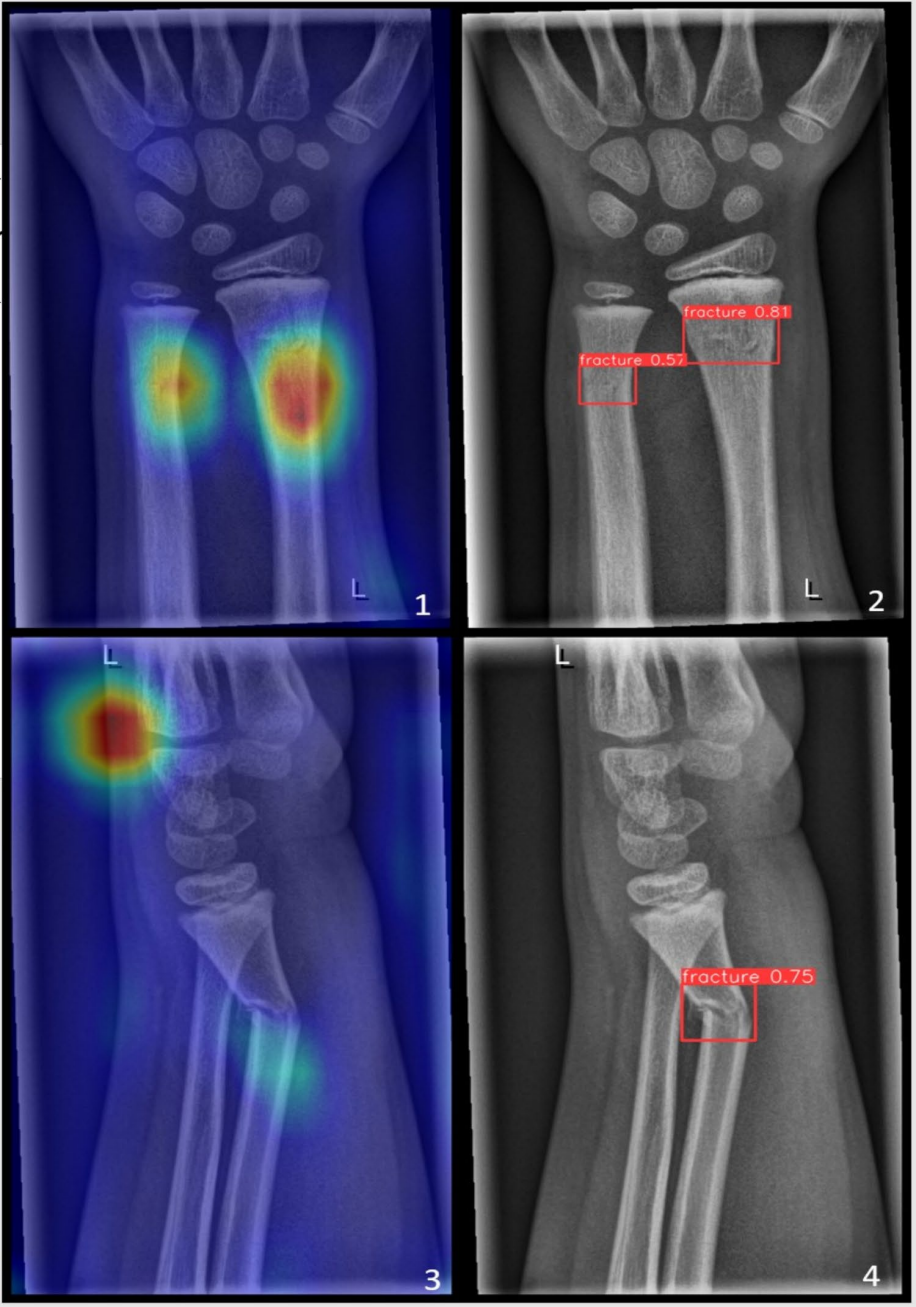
Figures 1 and 2 show that the existing fractures were predicted by both the EfficientNet image classification model in the first picture and the YOLOv8 object detection model in the second picture. The EfficientNet image classification model in picture 3 has incorrectly classified the X-ray of an obvious fracture as not being a *fracture*. Compared to a correctly detected fracture by the YOLOv8 object detection model in picture 4. Image from the ”GRAZPEDWRI-DX” dataset [23]
